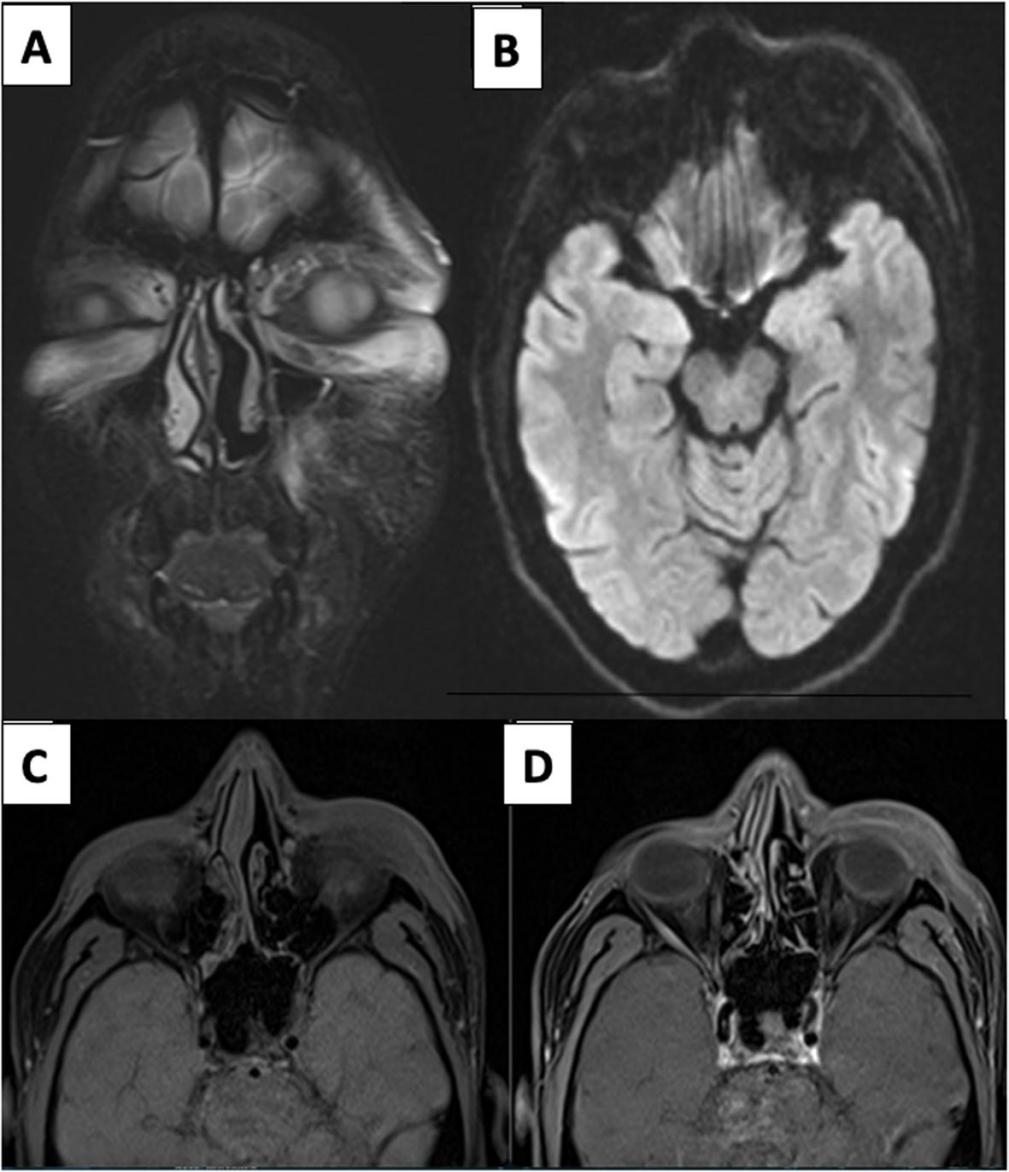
A Coronal fat sat T2-weighted images show bilateral (L>R) orbital hyperintensities involving preseptal, premalar fat, and frontal scalp tissue on the left side. B Axial DWI shows no evidence of diffusion restriction. C, D Pre- and post-contrast fat sat T1 axial images show no peripheral enhancement
